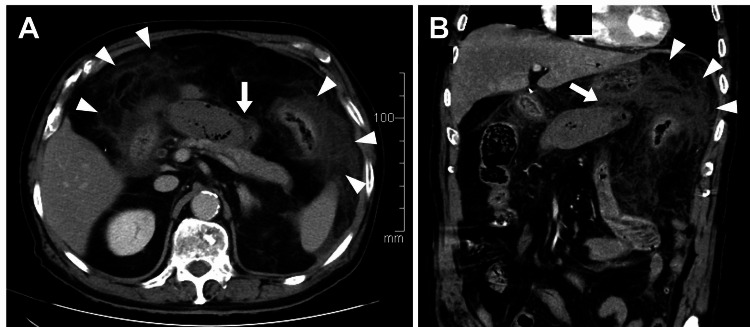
CT showing severe inflammation around the R-Y limb (A) Axial CT showing severe inflammation in the upper abdominal cavity (arrowheads), and the dilated tip of the R-Y limb (arrow). (B) Coronal CT showing severe inflammation in the left subdiaphragmatic region (arrowheads), and free air around the R-Y limb (arrow). CT, computed tomography; R-Y, Roux-en-Y
